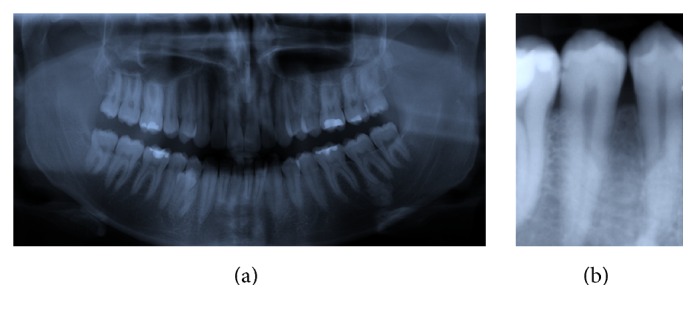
Case 3 radiography: ST in 4.4–4.5 region 2015 (a) and after extraction 2015 (b).
